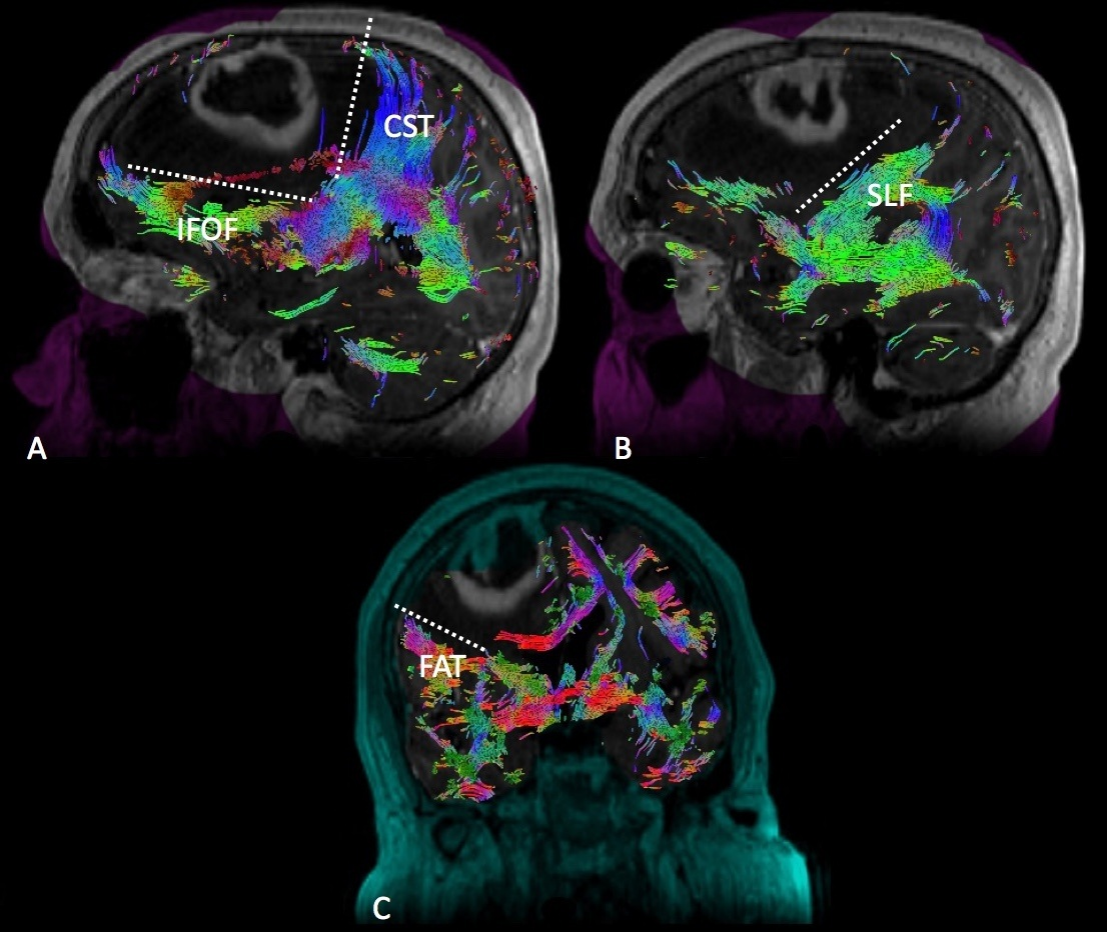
Medial Posterior Frontal Diffusion tractography enhanced T1 magnetic resonance imaging (MRI) with contrast with (A) sagittal, (B) sagittal, and (C) coronal views demonstrating a medial posterior frontal glioma in relation to the surrounding white matter tracts. Labeled white matter tracts associated are shown as follows: inferior fronto-occipital tract (IFOF), corticospinal tract (CST), superior longitudinal fasciculus (SLF), and frontal aslant tract (FAT). The disconnection pattern is shown as a white dotted line.
